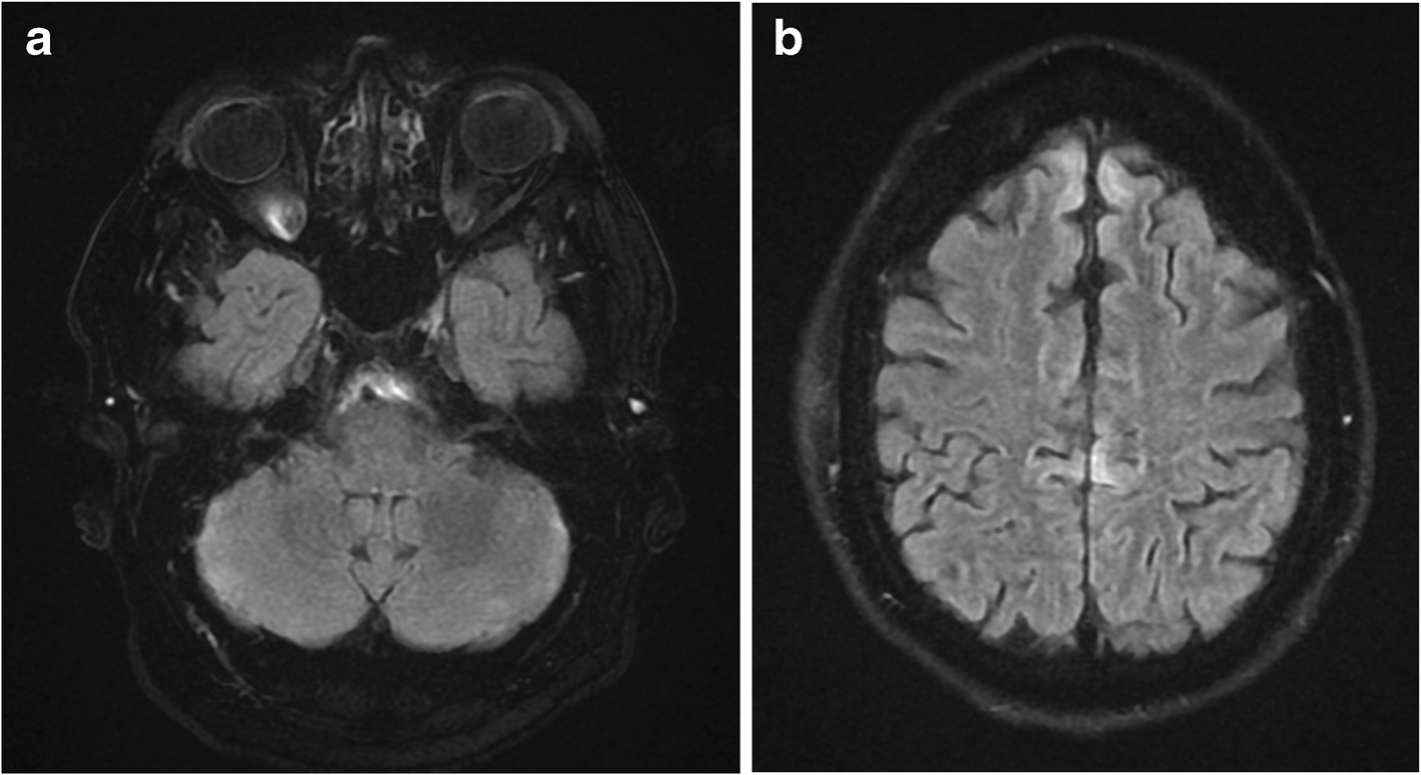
MRI after 6 weeks a and b displaying marked regression of the abnormalities.
